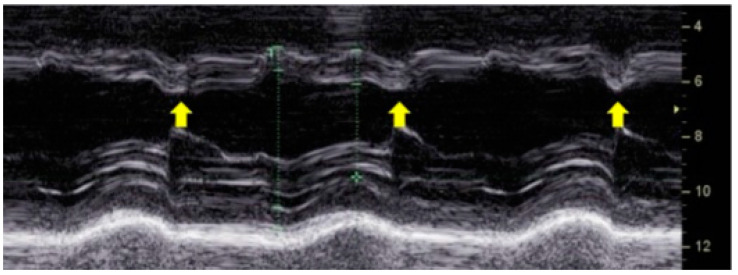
M-mode echocardiography showing septal flash, a characteristic sign of left bundle branch block. The yellow arrows indicate the early systolic septal motion abnormality caused by dyssynchronous ventricular activation.
